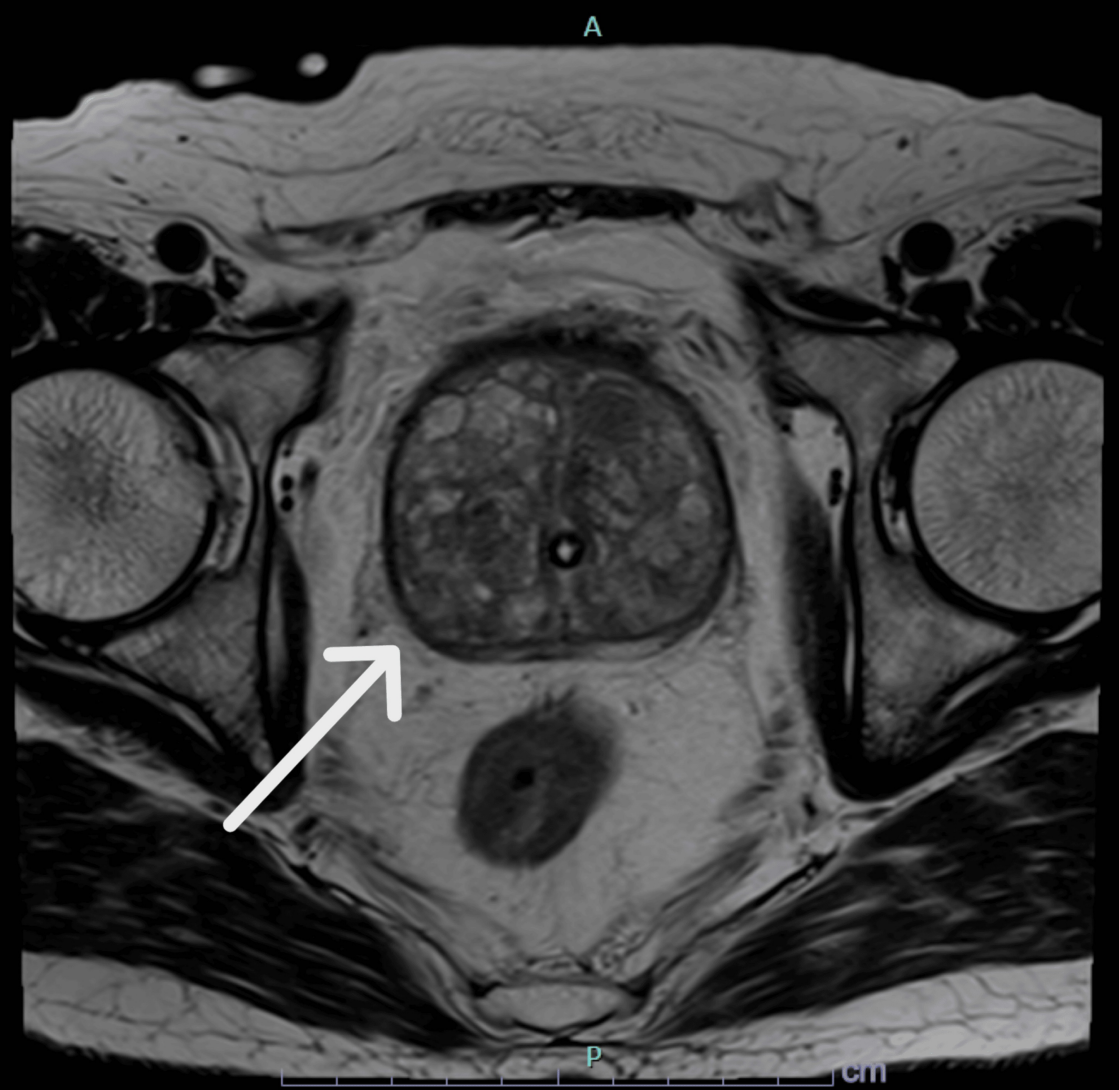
MRI T2 axial view of the massive prostate when a Foley catheter was placed
